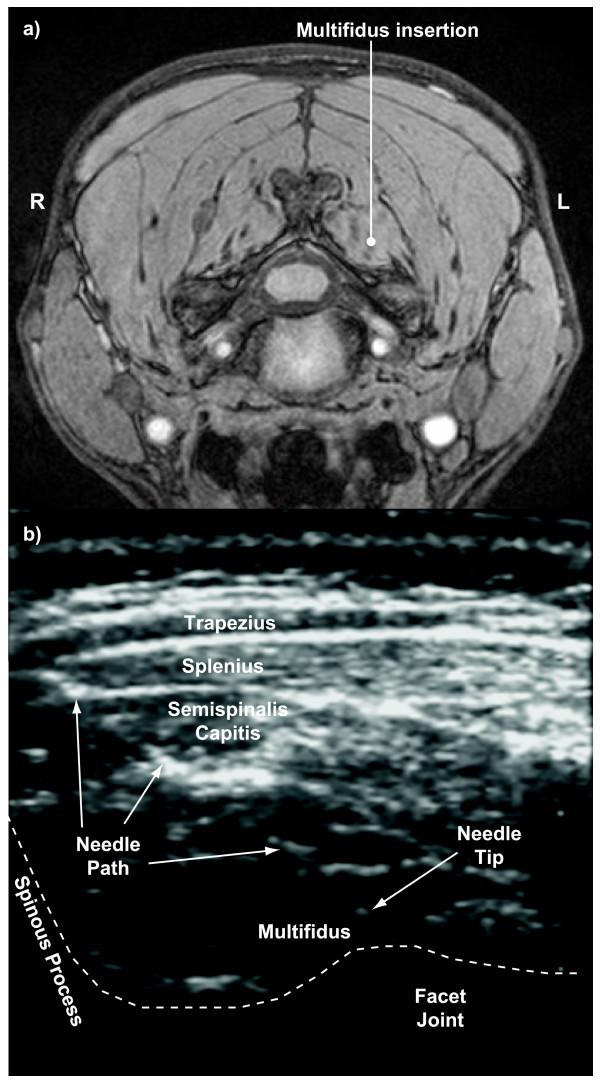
MRI and ultrasound images showing wire insertion. a) axial slice of a magnetic resonance scan at C4 level showing desired insertion path to the multifidus muscle and b) ultrasound image showing actual needle path and tip during insertion into the multifidus muscle.
